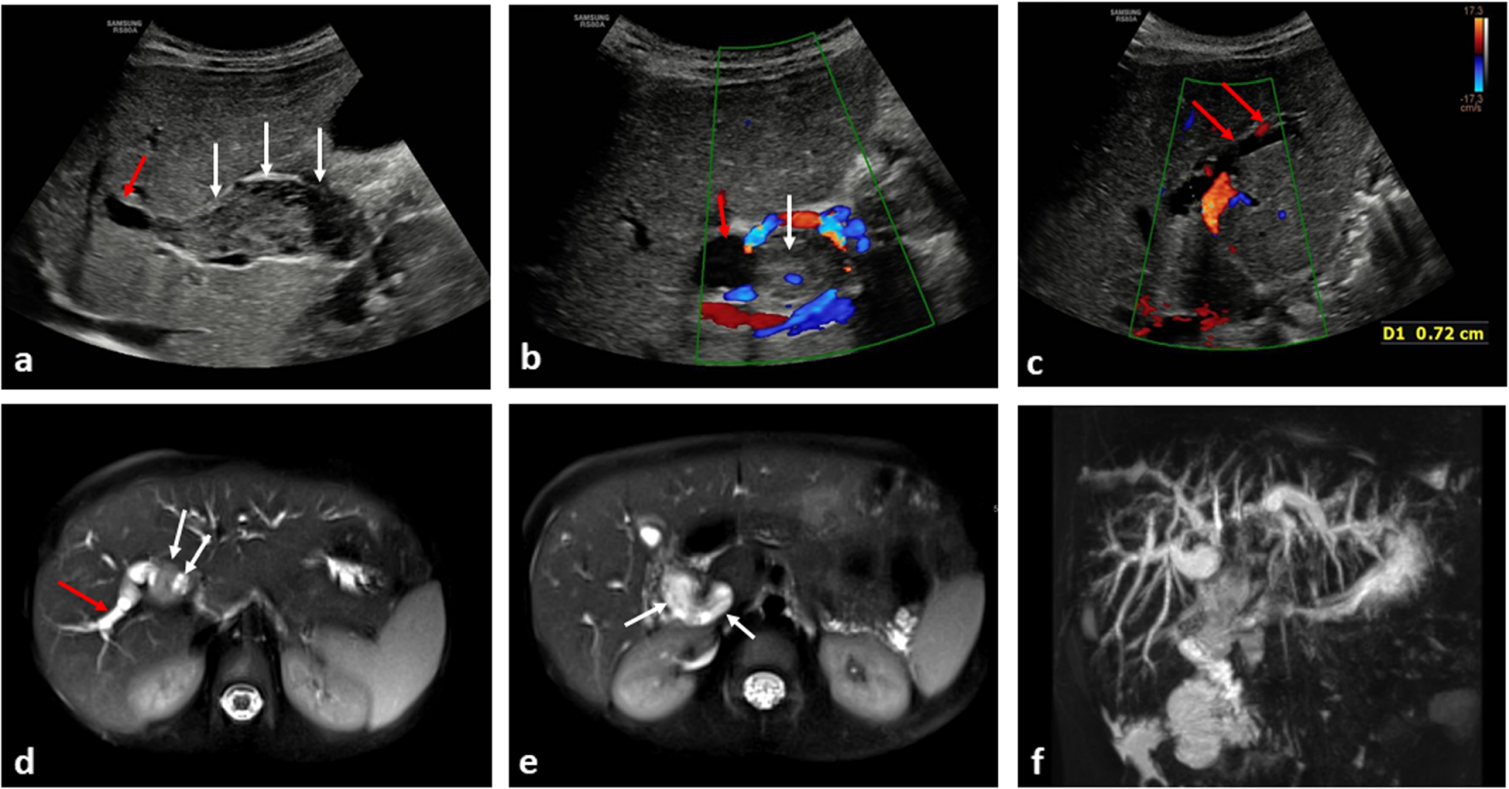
A 5-year-old boy presenting with jaundice from biliary rhabdomyosarcoma. a Longitudinal and (b, c) transverse ultrasound images of the liver at diagnosis, demonstrates a heterogenous mass (white arrows) occupying and distending the common bile duct with downstream intrahepatic biliary dilatation (red arrow). d, e Axial T2-weighted MRI images through the liver confirm the intra-biliary mass (white arrows), with dilatation of right-sided intrahepatic bile ducts (red arrow). f The intrahepatic biliary dilatation is best demonstrated on the 3D-maximum intensity projection (MIP) image of the MRCP (MR cholangiopancreatography)
